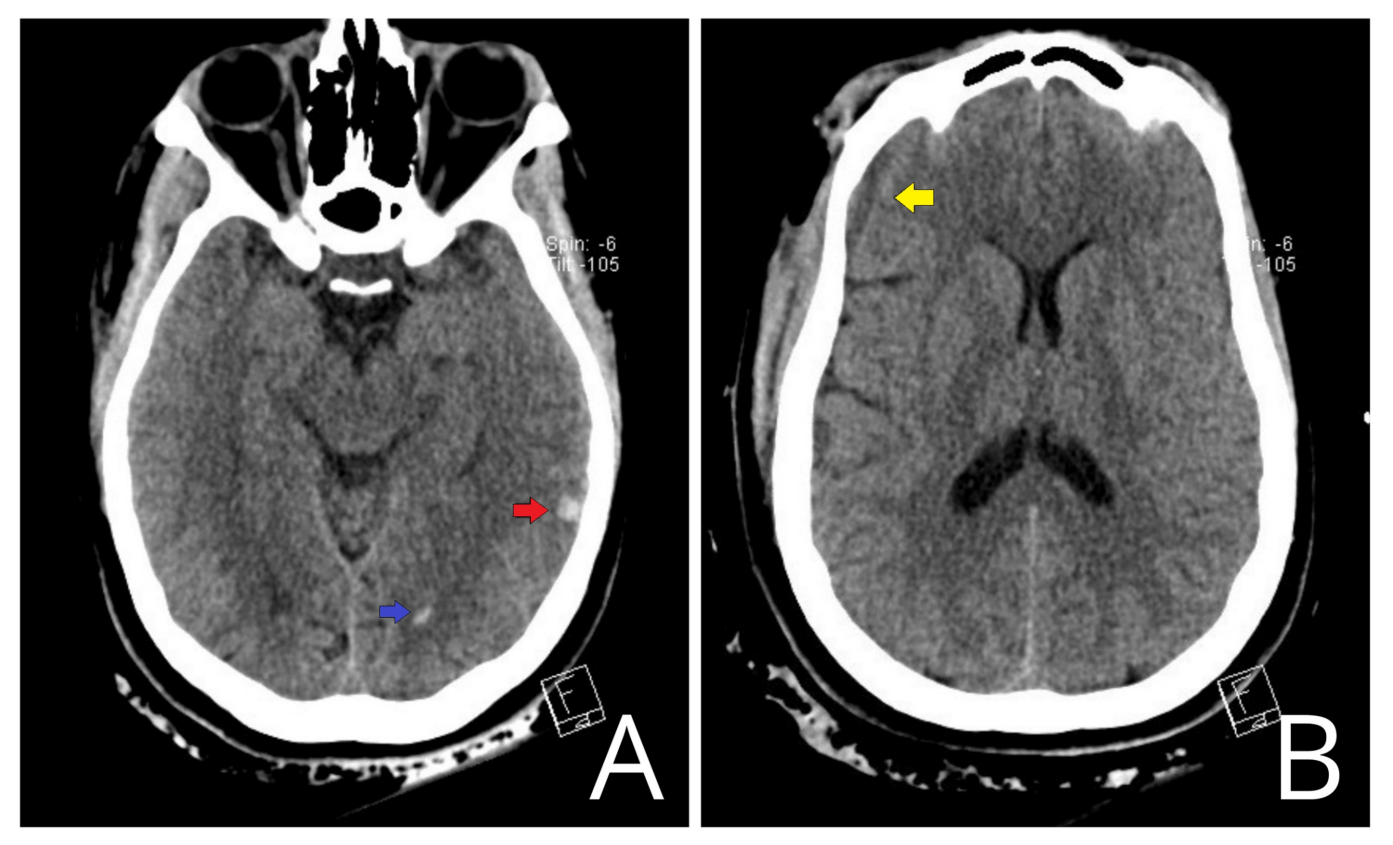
CT head of patient 2 Axial non-contrast CT of the head. (A) Temporal lobe contusion (red arrow) and intraventricular hemorrhage (blue arrow). (B) Trace subdural collection (yellow arrow). On neurological examination, the patient presented with eyes closed to pain, pupils 5 mm on the right and 4 mm on the left, both reactive to light, non-verbal, and extensor posturing in all extremities. CT: computed tomography
